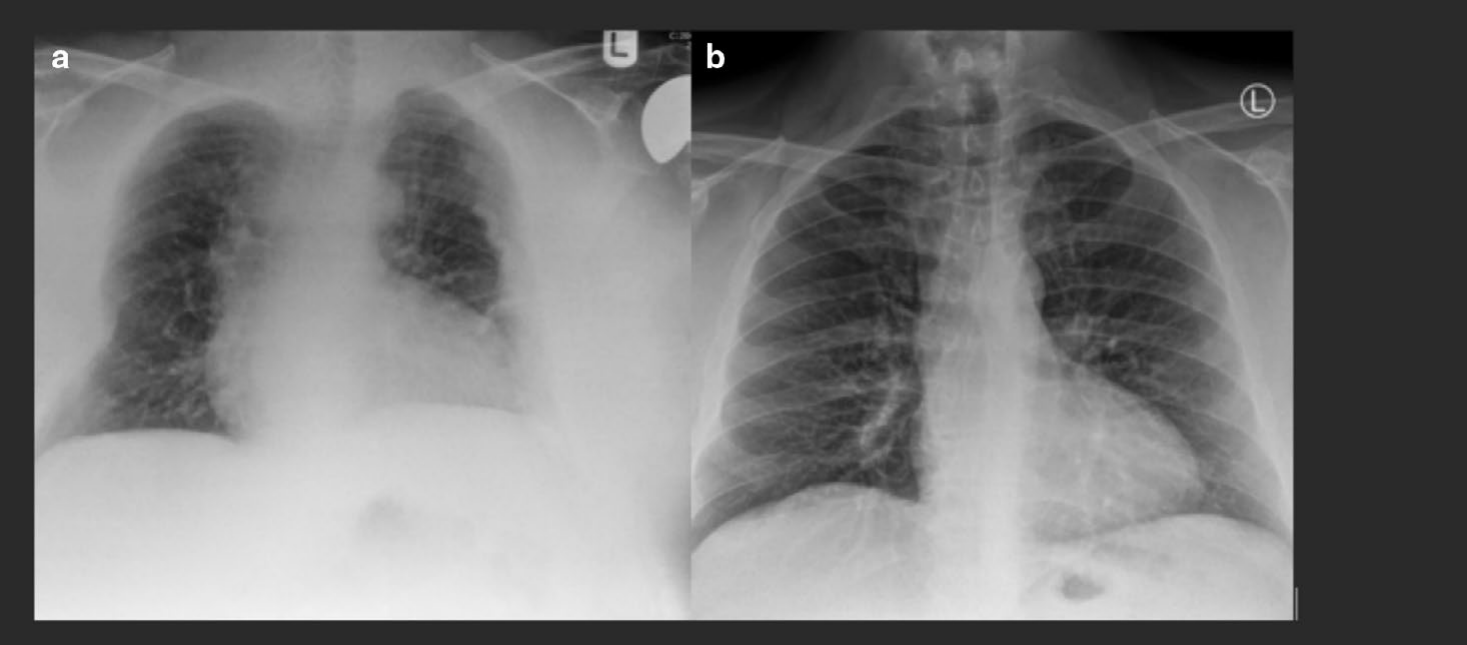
(a, b) Chest radiographs of the patient at time of presentation (left) and 2 years prior (right), demonstrating features detailed below.
